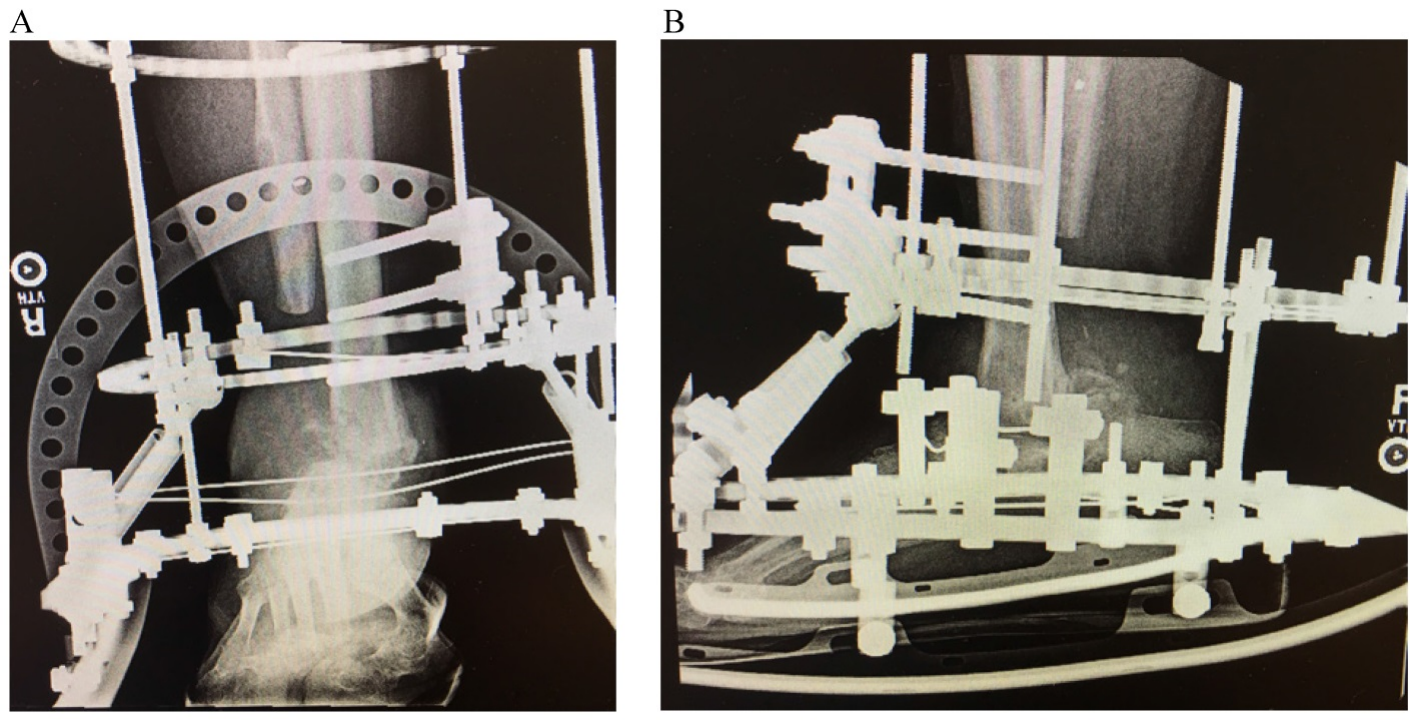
AP (A) and lateral (B) radiographs following definitive ankle fusion after eradication of infection
